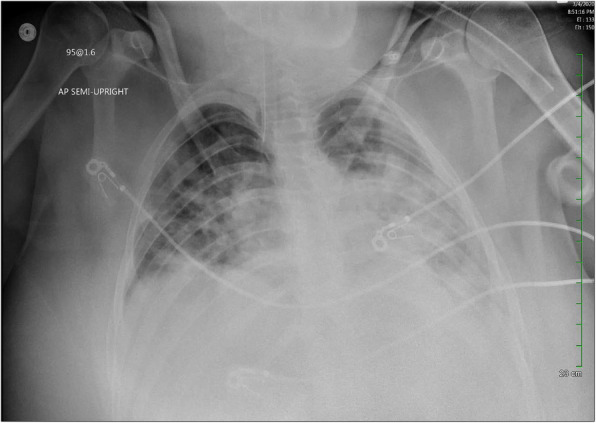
Chest radiograph obtained on day 1 of admission to intensive care unit revealed bibasilar airspace disease
